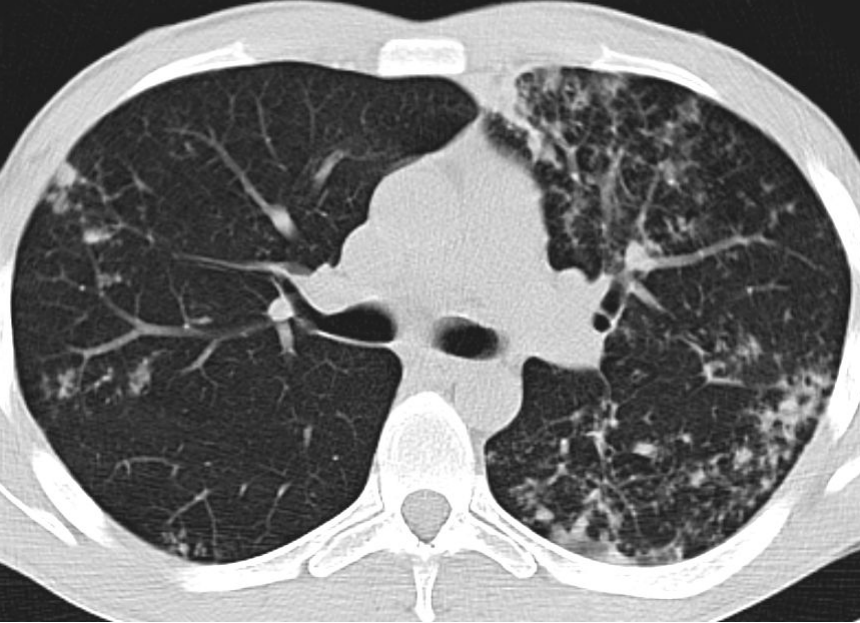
28 year-old man with pulmonary tuberculosis, HRCT shows bronchogenic spread and interstitial changes with peribronchial thickening.
